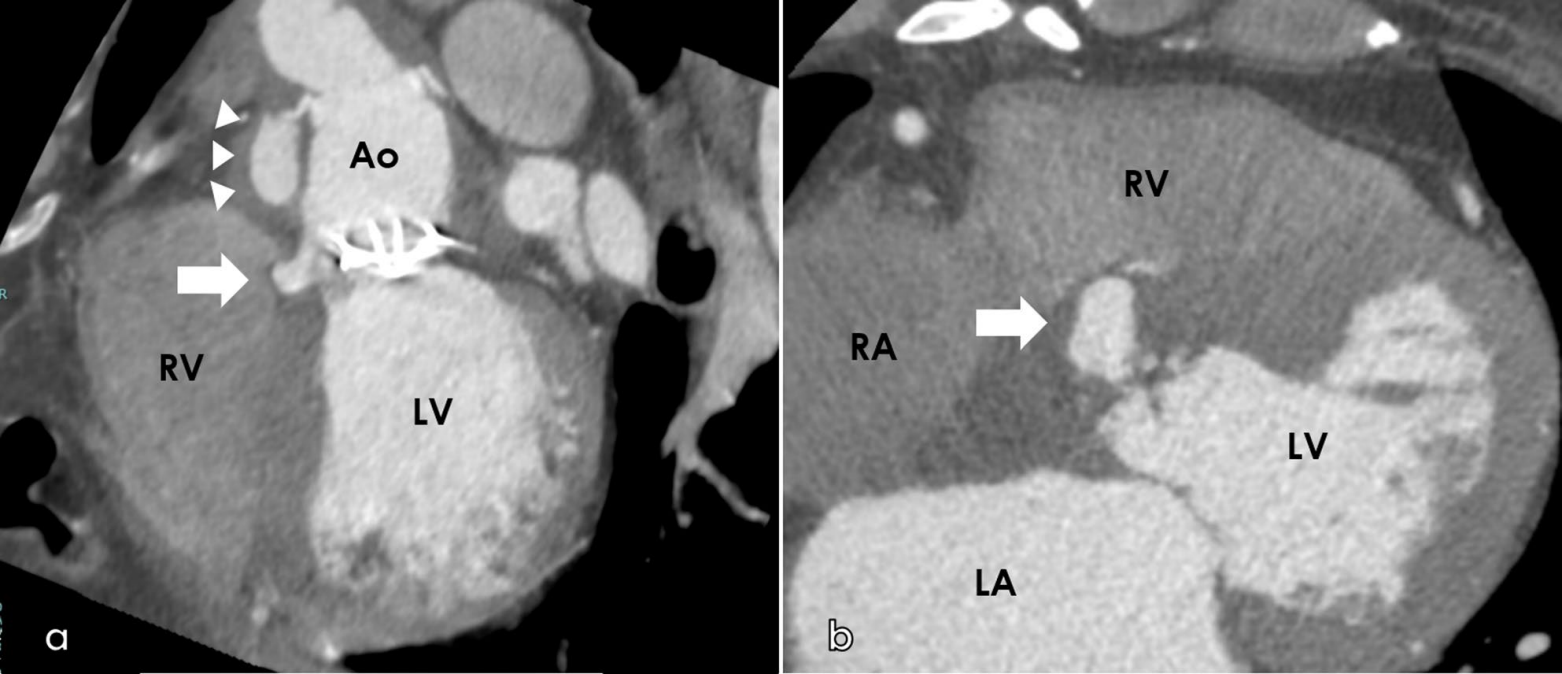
Cardiac computed tomography showing the formation of a periannular abscess extending into the ventricular septum (a) Long-axis view. A periannular cavity (white arrow) is visible at the level of the right coronary cusp of the aortic valve, extending deeply into the ventricular septum toward the right ventricle. A pseudoaneurysm is also observed at the proximal anastomosis site following hemiarch replacement (white arrowhead) (b) Short-axis view. The periannular abscess (white arrow) encroaches upon the ventricular septum, demonstrating its deep extension into the septal tissue *Ao* ascending aorta, *RA* right atrium, *LA* left atrium, *RV* right ventricle, *LV* left ventricle
